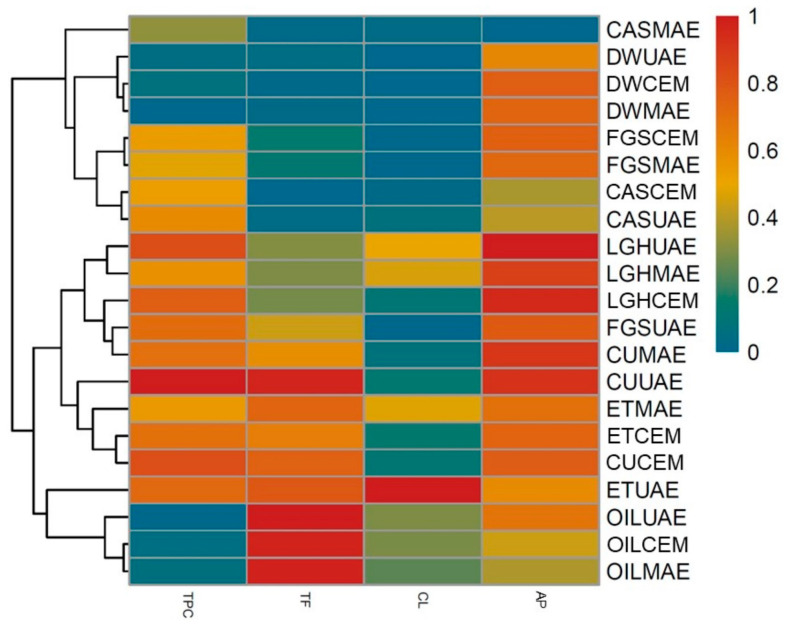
Heatmap and dendrogram of the total phenolic content (TPC), total flavonoid (TF), total chalcones (CL), and antioxidant power (AP) of extracts obtained with conventional and non-conventional method. MAE: microwave-assisted extraction; UAE: ultrasound-assisted extraction; CEM: conventional extraction; DW: distilled water; ET: ethanol; NaDESs: LGH (lactic acid: glucose), CAS (sucrose: citric acid), CU (choline chloride: urea) and FGS (fructose: glucose: sucrose).
